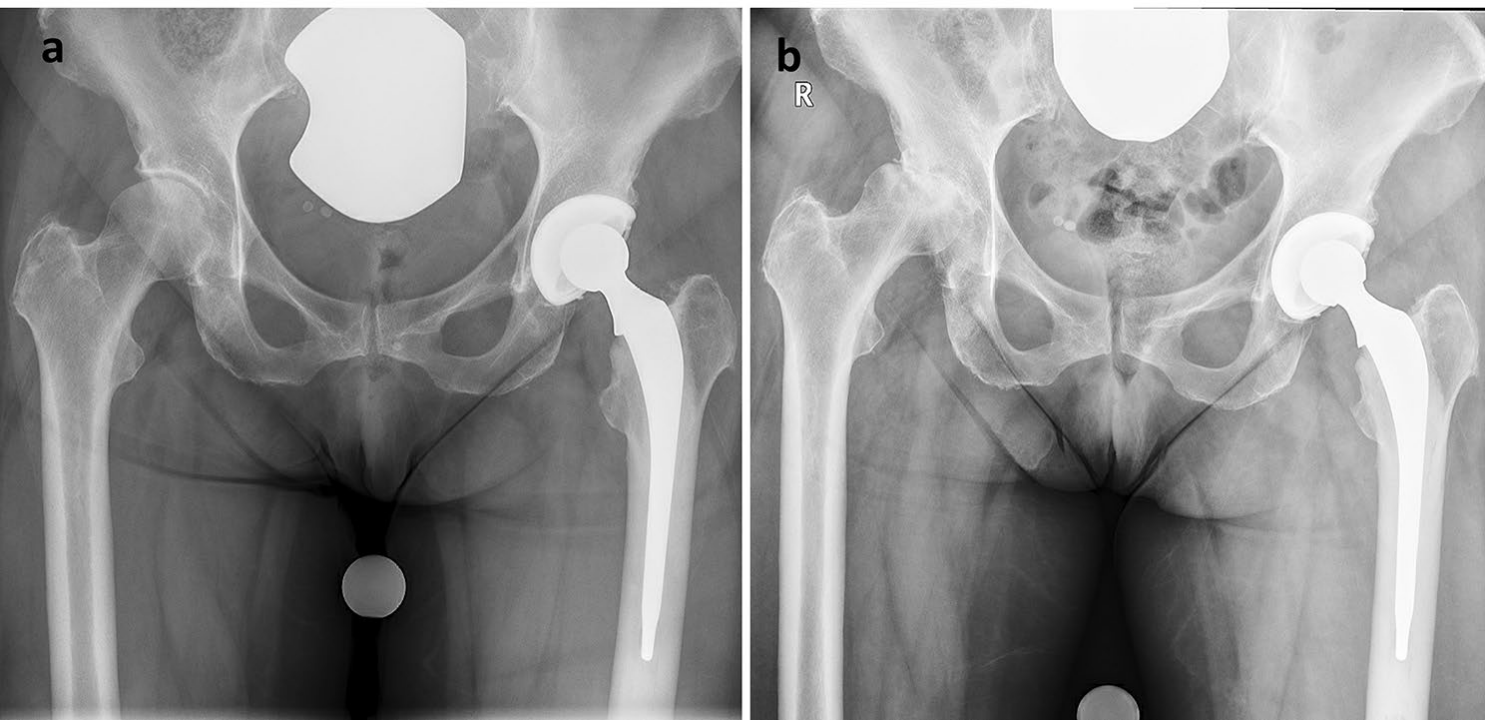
Radiographs of the pelvis of a 71-year-old female in antero-posterior view. (a) Radiograph prior to ICSI to the right hip: The right hip shows joint space narrowing, subchondral sclerosis and initial osteophytes. A total hip replacement with cemented stem is present on the left side, the arthroplasty is in correct position with no signs of loosening. (b) Radiograph 4 months after ICSI to the right hip. The left hip shows RPIO with bone-to-bone OA and severe bone loss and off-centered femoral head
